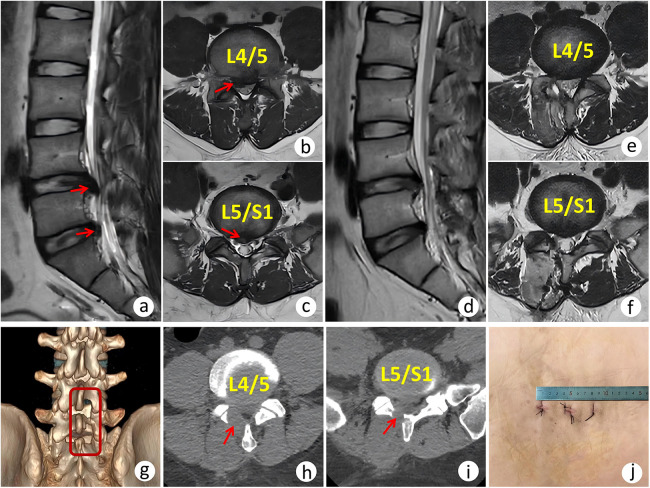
A 35-year-old male patient with L4/5 and L5/S1 AT-LDH treated by UBE. (a–c) Preoperative MRI demonstrating disc herniation at L4/5 and L5/S1 levels (arrow). (d–f) Postoperative MRI demonstrating complete removal of the herniated disc. (g) Postoperative 3D reconstruction demonstrating partial laminectomy at L4 and L5 levels (boxed area). (h–i) Axial CT scan demonstrating laminar fenestration (arrow). (j) Postoperative incision length.
